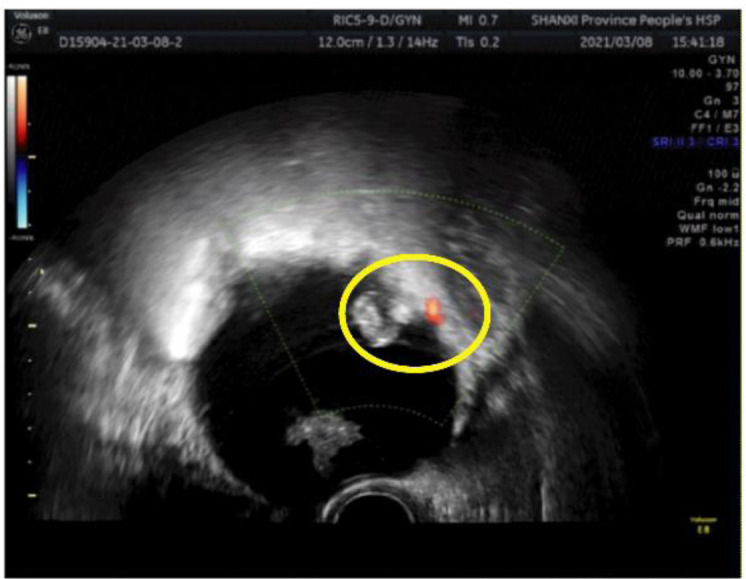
45-year-old patient with cystic lesion of the right ovary with 360° torsion. The cyst wall is lined with cuboidal epithelium. Less thyroid follicles can be seen in the focal cyst wall, and the boundary is clear. A hyperechoic mass can be seen inside, and the capsule wall can be seen by color Doppler. The yellow circles represent the dotted blood flow signal on the cyst wall.
